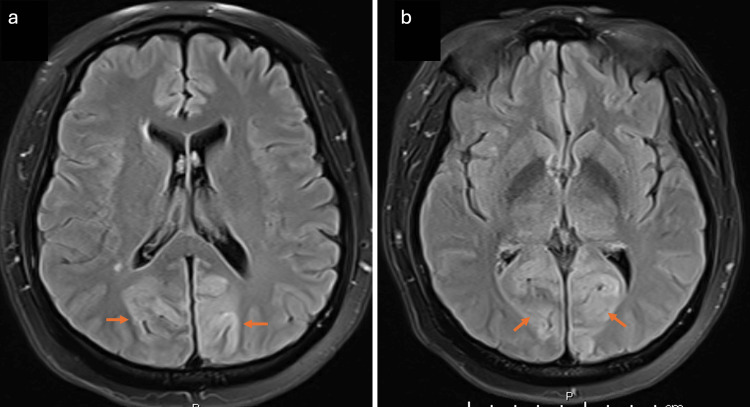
MRI of the brain. FLAIR images show areas of high signal intensity (arrows) involving the cortical and subcortical white matter regions of the bilateral parietal lobe (a) and occipital lobe (b). FLAIR: Fluid-attenuated inversion recovery
